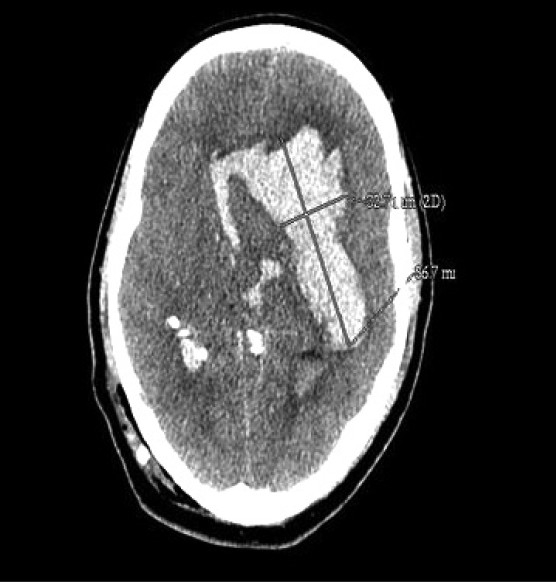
Postoperative CT scan of brain showing massive intracerebral bleed
